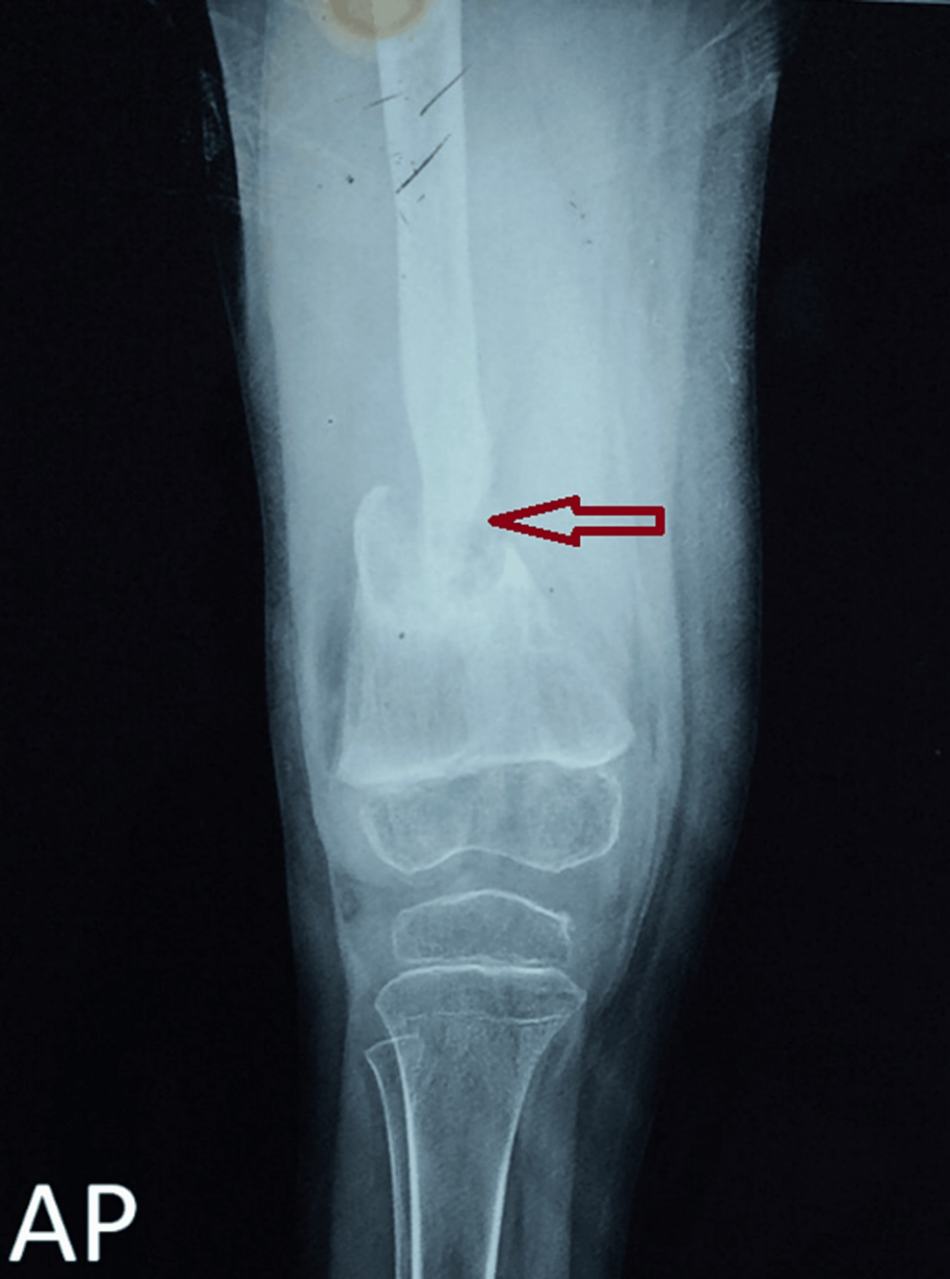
A plain radiograph (AP view) of the right distal femur with the right knee joint showing pathological fracture of the distal one-third of the right femur
